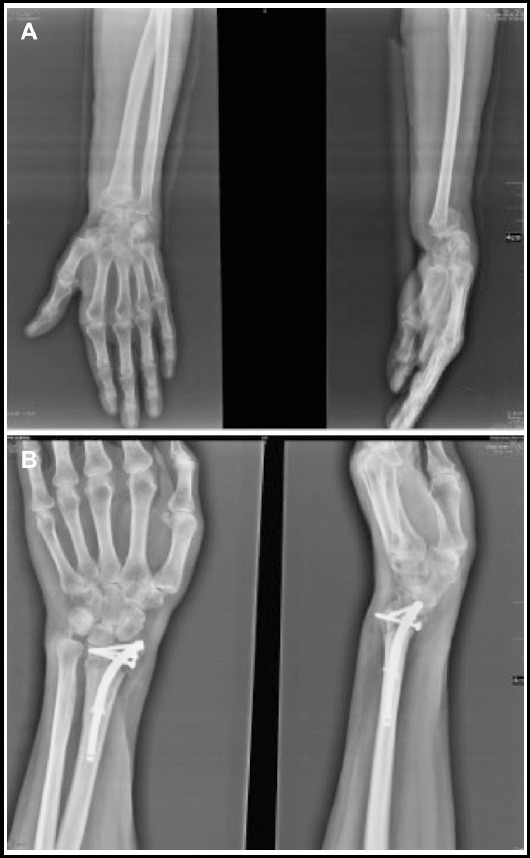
Anteroposterior and lateral radiographics of distal radius fractures before (a) and after (b) internal fixation with intramedullary nailing device Sonoma Wrx.
